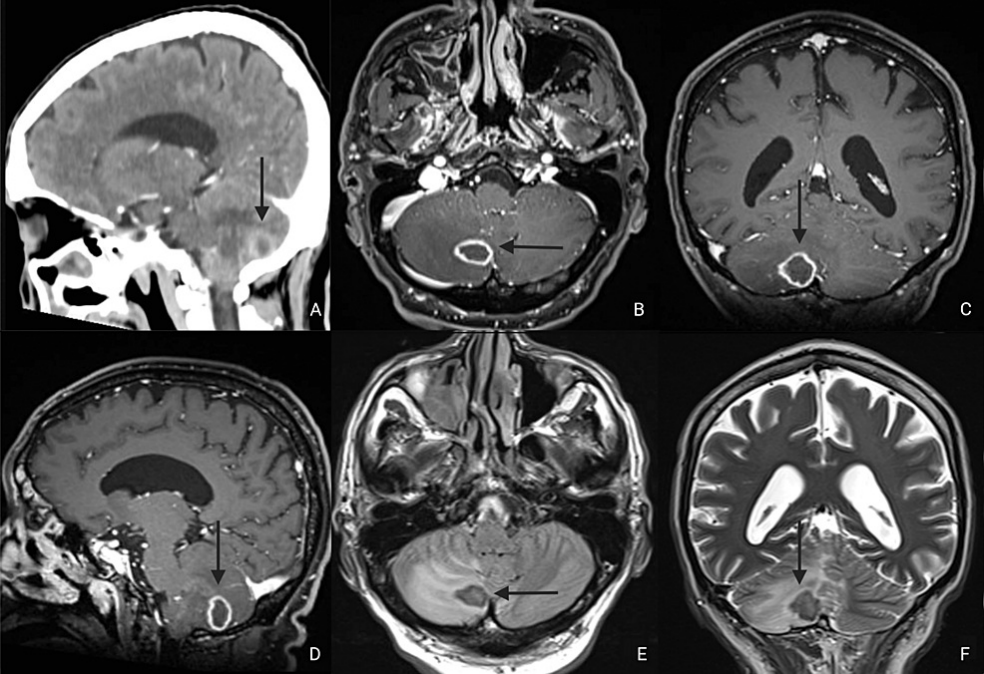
CT (A) and MRI (B-F) A: Sagittal CT with contrast showing a ring-enhancing lesion at the suboccipital cerebellar surface. B, C, D: Axial, coronal, and sagittal T1-weighted contrast imaging demonstrates an ovoid ring-enhancing lesion with a thickened subcapsular appearance in the right cerebellar hemisphere. E: Axial FLAIR showing surrounding cerebellar edema. F: Coronal T2-weighted image shows a hypointense cerebellar paravermian lesion. FLAIR: fluid-attenuated inversion recovery
